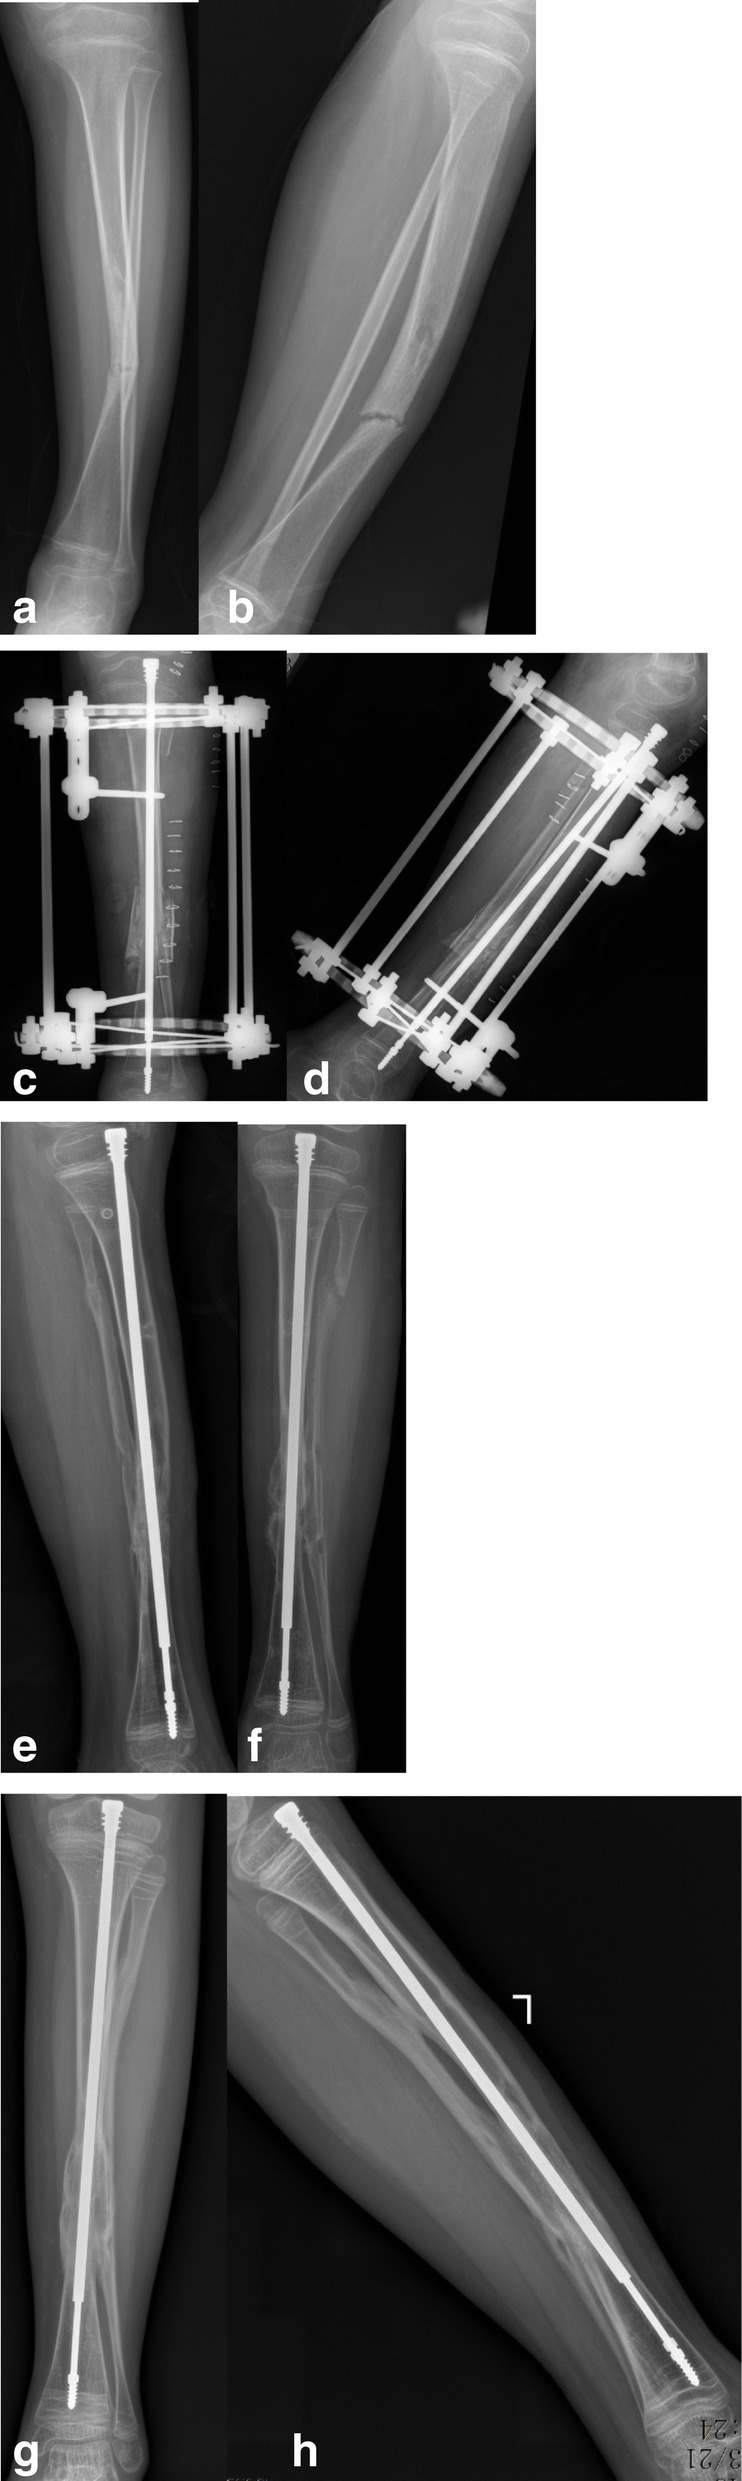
a, b A 6-year-old girl. Preoperative anteroposterior and lateral radiographs. c, d The X-ray image at 1 week of post-operation. e, f Seven months after the operation. g, h Sixteen months after the operation with telescopic rod displacement
